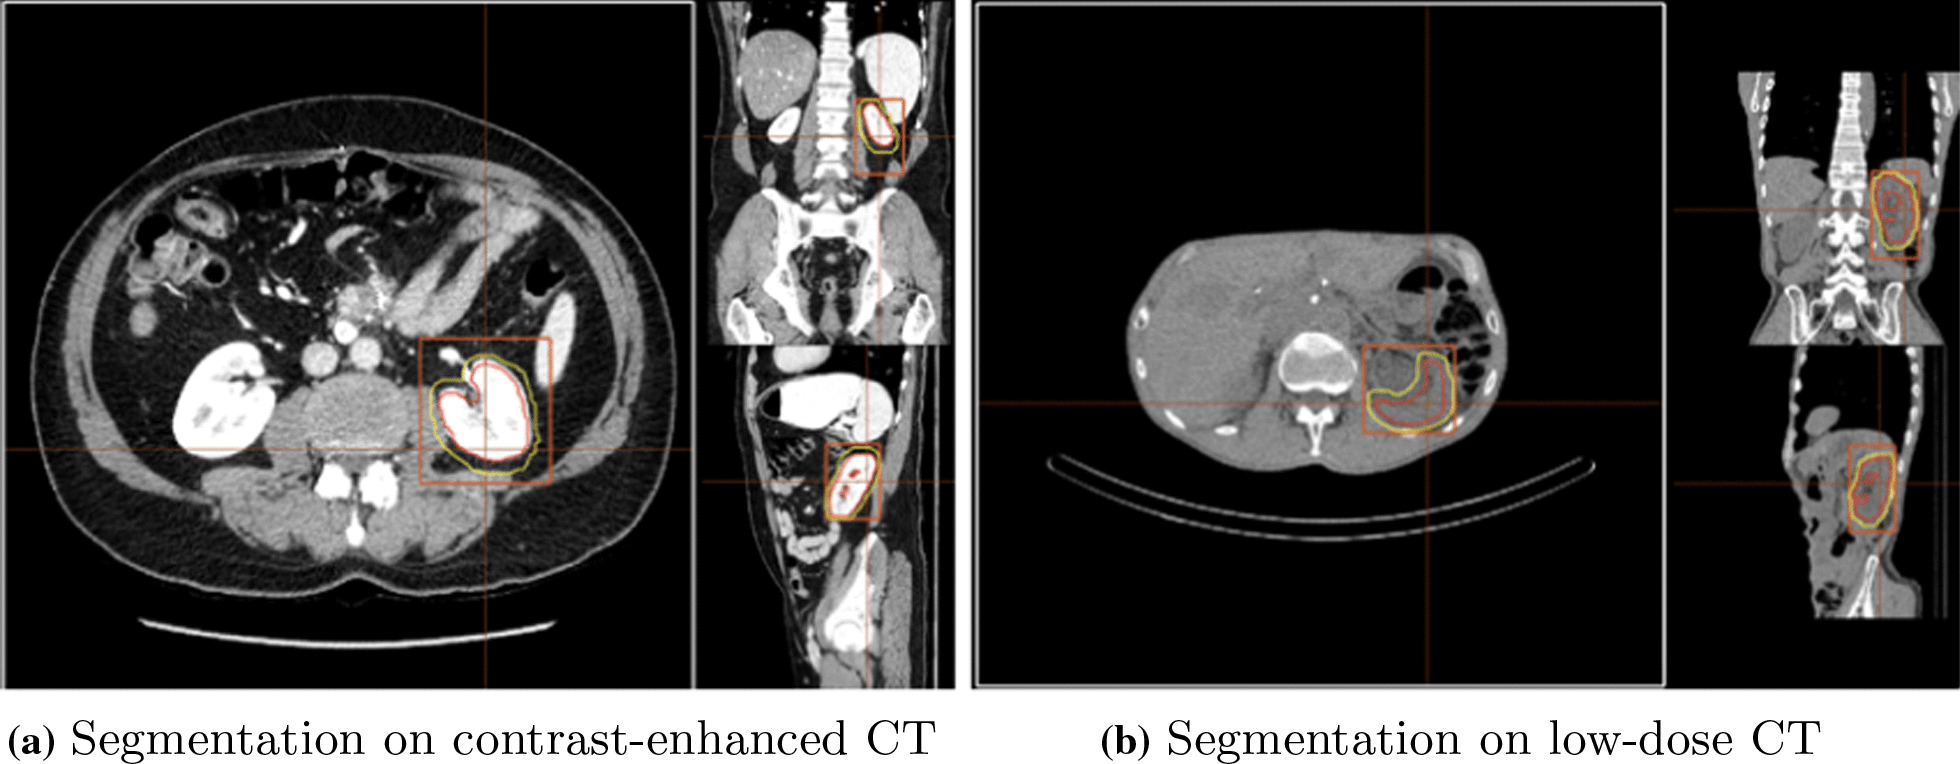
Segmented left kidney along axial, sagittal and coronal axis using the AI . The segmentation boundaries are highlighted with red contour on a contrast-enhanced CT on the left-hand side and on a low-dose CT on the right-hand side. The red rectangle corresponds to the bounding box used in kidney detection by the algorithm and the yellow contour is the 3 mm expanded region for activity retrieval from the SPECT images based on the CT-segmentation
